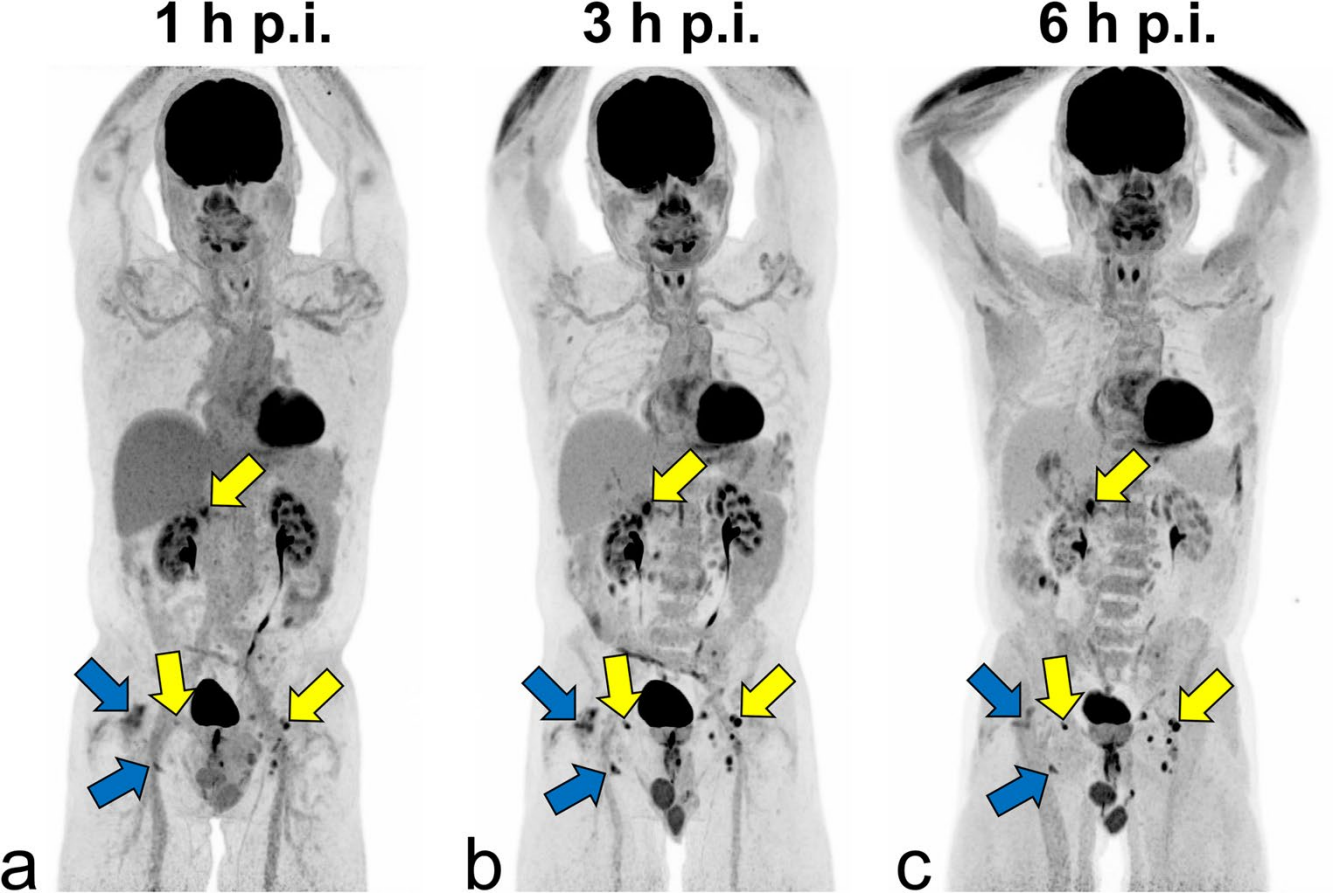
Maximum intensity projections of [¹⁸F]FDG PET/CT (3 MBq/kg) in a 74-year-old patient with newly diagnosed follicular lymphoma (grade 1–2), acquired at 1 h (a), 3 h (b), and 6 h (c) p.i. Multiple pathologically enlarged lymph nodes are observed in the retroperitoneal and bilateral inguinal regions. At 1 h p.i., some lymph nodes show moderately increased metabolic activity (yellow arrows), while others appear metabolically inactive (blue arrows). Delayed imaging at 3 h and 6 h p.i. reveals progressive [^18^F]FDG accumulation in all marked lymph nodes with improved TBR, alongside decreasing uptake in parenchymal organs and the blood pool. Additionally, increased [^18^F]FDG uptake in the bone marrow and skeletal muscles is noted on the late-phase scans
